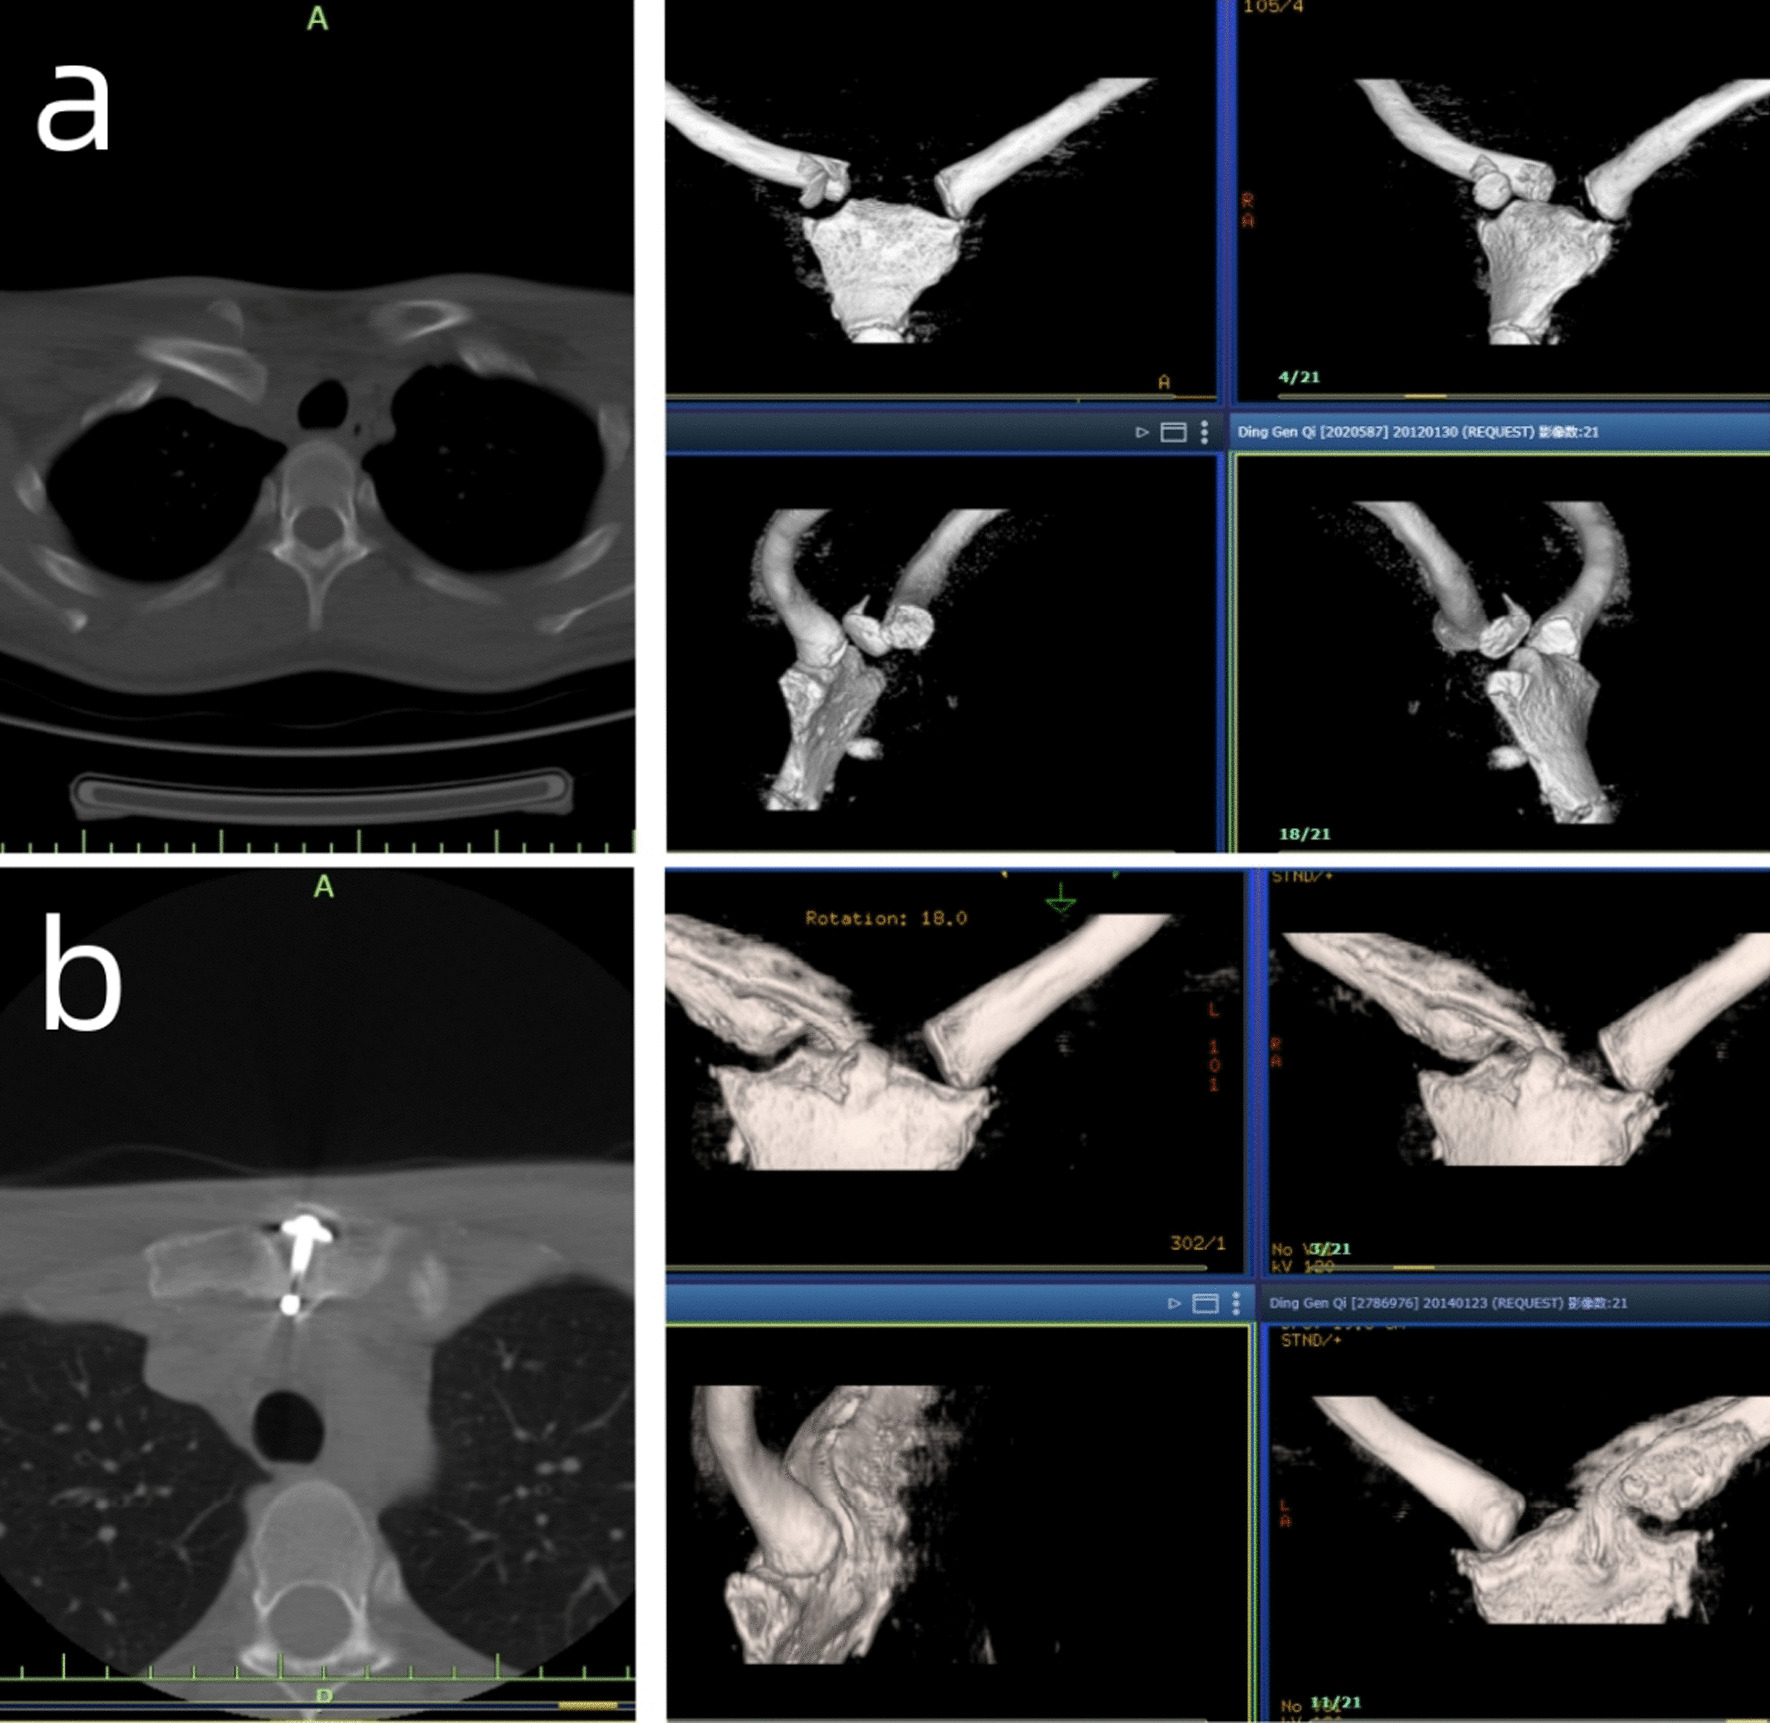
a CT image of a 33-year-old male patient with posterior dislocation of left sternoclavicular joint preoperatively; b The plate and joint was restored to normal 2 years postoperatively
